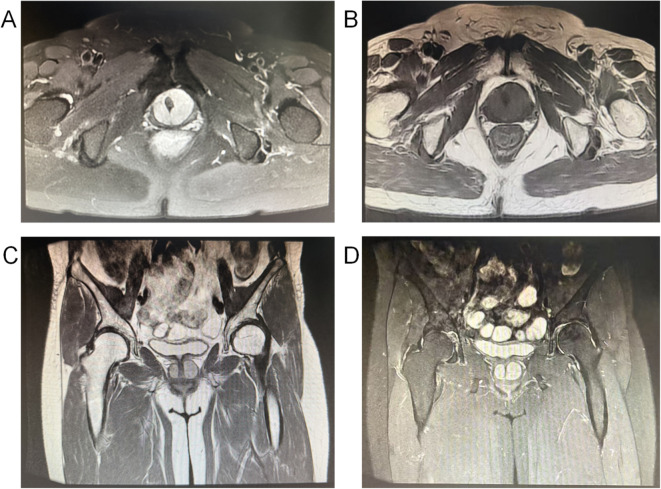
(A) is a Axial T2-weighted fat suppression sequence image, (B) is a Axial T1-weighted image, (C) is a Coronal T2-weighted images acquired using “in-phase” technology with high-homogeneity fat suppression, (D) is a Coronal T2-weighted images acquired using “water-induced” technology with high-homogeneity fat suppression. MRI sagittal T2-weighted image shows a round lesion with isointense T1 signal and hyperintense T2 signal in the perurethral and anterior vaginal wall regions. The lesion exhibits heterogeneous internal signal intensity with well-defined margins and shows heterogeneous contrast enhancement on contrast-enhanced imaging.
